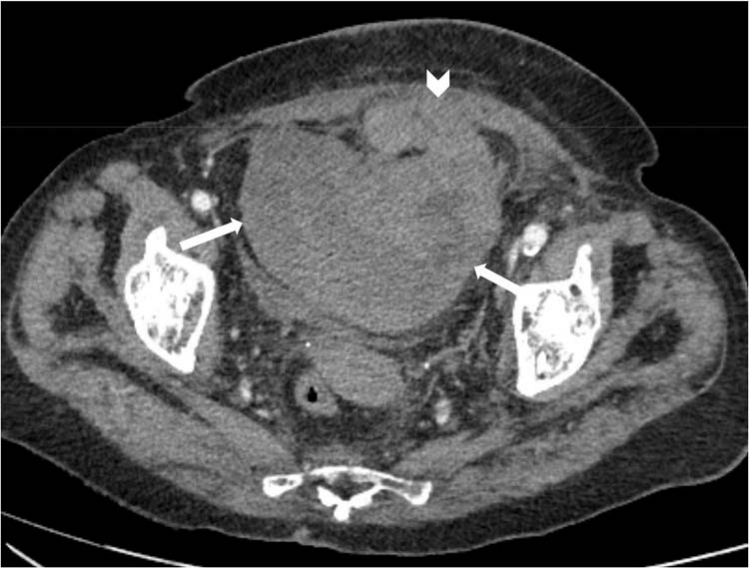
Axial non-contrast CT abdomen and pelvis at the level of acetabulum. Rectus sheath haematoma (chevron) that appears to have dissected through the rectus sheath into the extraperitoneal pelvic space (arrows).
